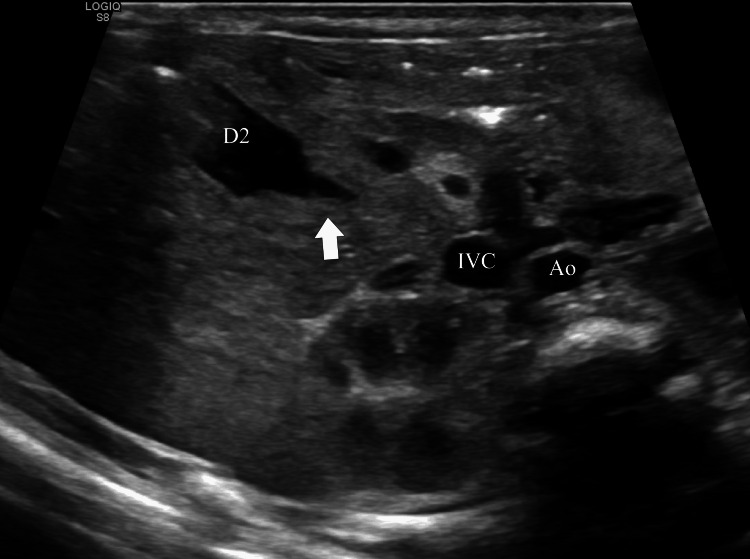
Gray-scale ultrasound plane demonstrating a rim of pancreatic tissue around the duodenum Gray-scale oblique image shows a hyperechogenic band of pancreatic parenchyma encircling the distal portion of the descending duodenum. A beaked appearance of the contour of the distal prestenotic duodenum (arrow) is noted as it enters the pancreatic head. Ao, Aorta; D2, Descending duodenum; IVC, Inferior vena cava
